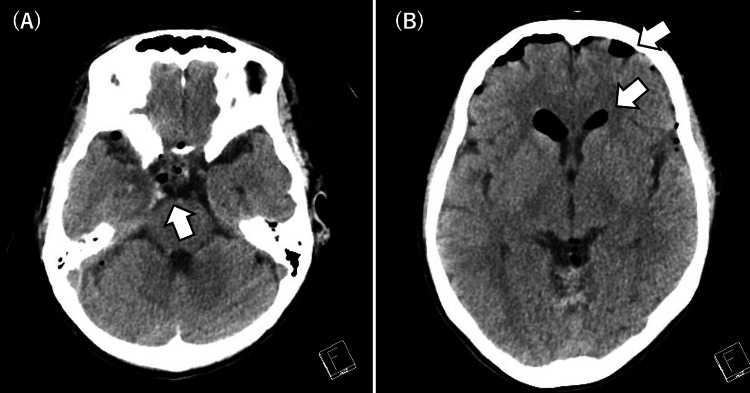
Head CT on post-injury day 1. A: Decreased air in the suprasellar cistern (arrow). B: Decreased air in the ventricles and on the cortical surface of the brain (arrow).
